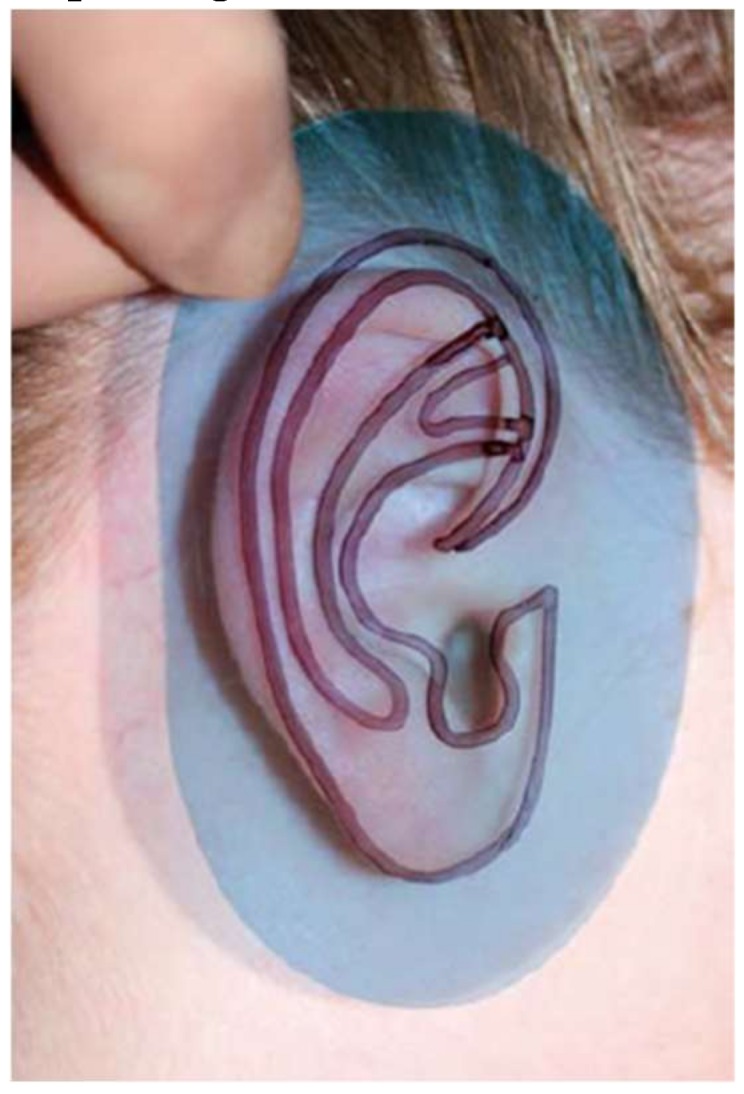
2D template of the ear. A translucent X-ray film is placed against the opposite ear and the main’s shape features are hand-traced, creating a 2D projection of the 3D shape.
